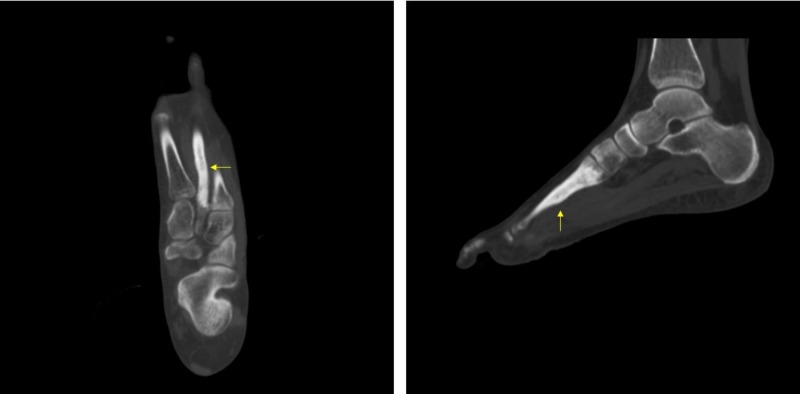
Pre-operative computed tomography (CT) showing cement in the second metatarsal.
